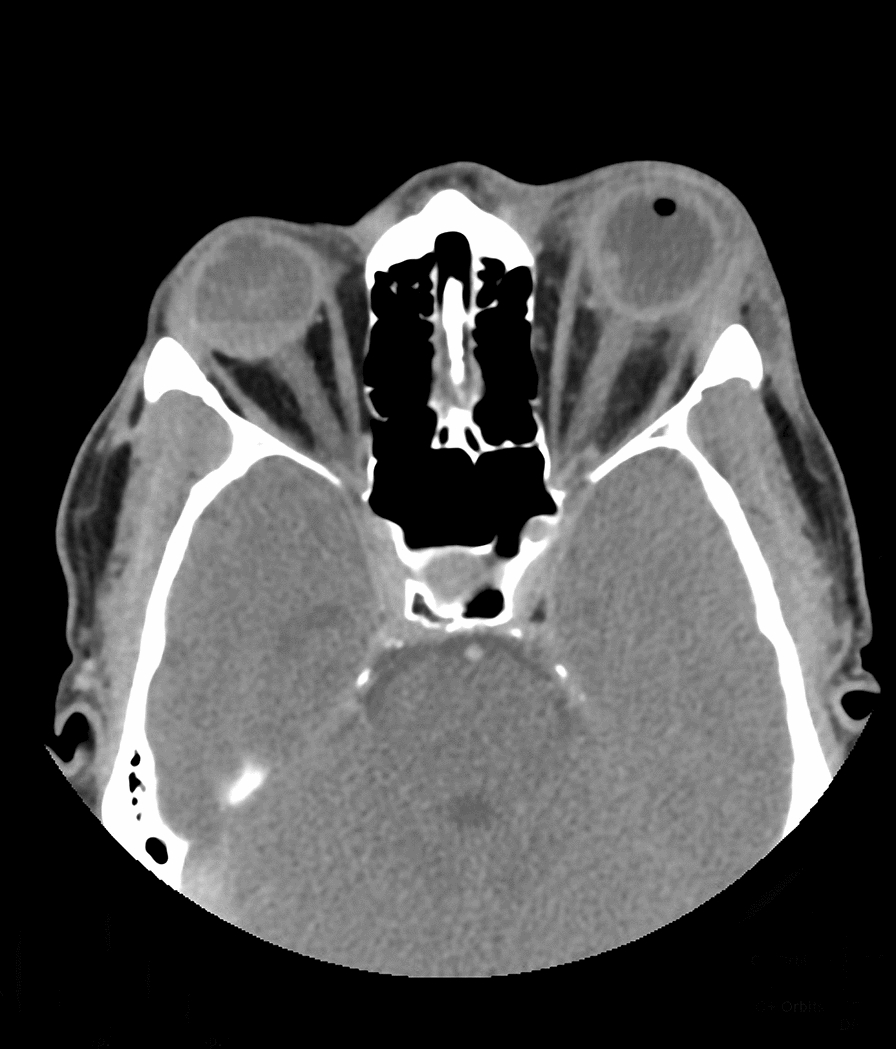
Computerized tomography (CT) of orbit revealed diffuse preseptal thickening with evidence of extension along the episcleral and proximal part of the optic nerve with infiltration of the tendinous space associated with diffuse thickening along the choroidoretinal thickening
